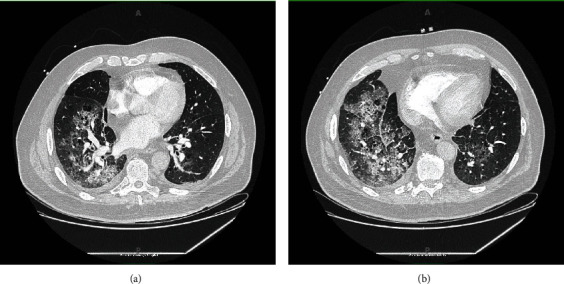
(a, b) CTA chest showing airspace opacities in the right upper lobe and right lower lobe as well as minimally in the left lower lobe, small pleural effusions bilaterally.
